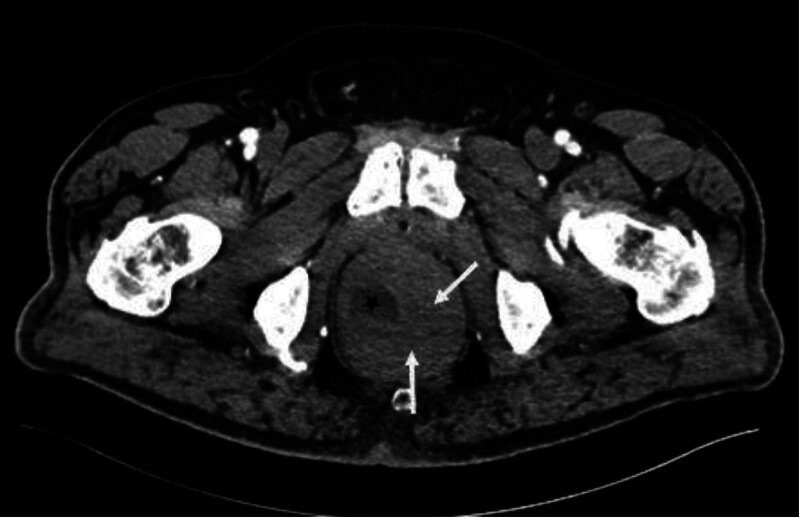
Large rectal hematoma (white arrows) visible on computed tomography image. The lumen of the rectum is marked with a black star.
